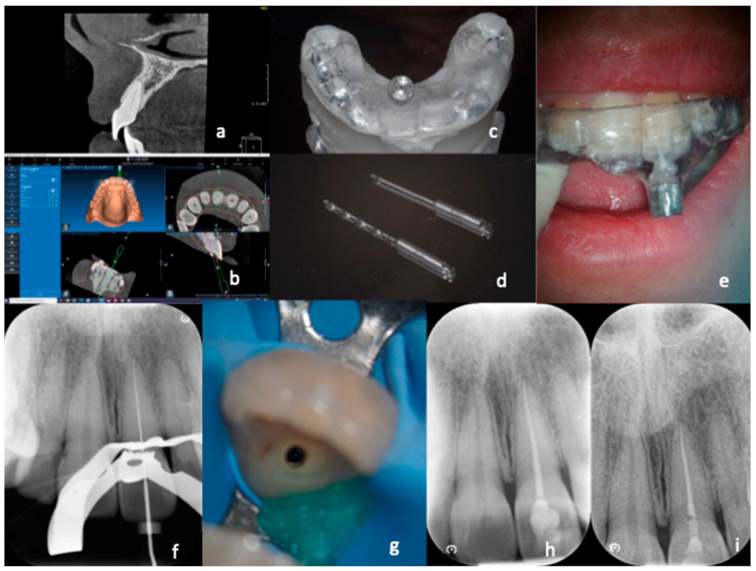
Treatment with “optimal precision” of a calcified canal. After carrying out a small FOV CBCT (a), which highlights the calcification of the canal, the DICOM data of the CBCT are coupled with the STL data derived from the digital impression (b). The result is a digital project that enables creation of a guide (c) which allows insertion of the two drills with a diameter of 0.9 mm up to the desired depth to reach the canal (d). The guide is inserted (e) and proceeds, with short excursion movements, to reach the canal. After rubber dam application, the working length is determined (f). Dentin removal is reduced even in the most coronal segment (g). Photos from the end of the treatment revealed correct obturation of the root canal system (h). A control X-ray was taken after six months (i).
